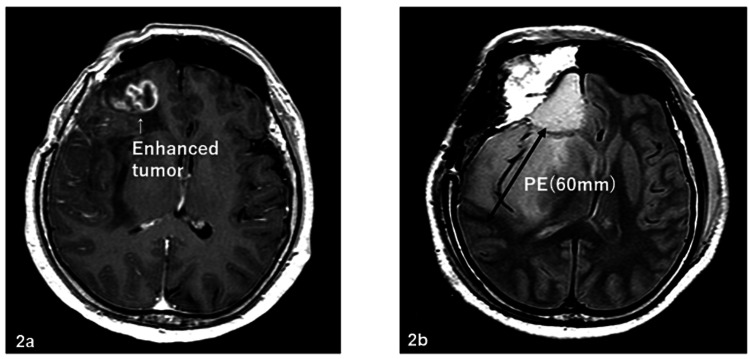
Supplementary MRI images – 2 2a: Gd-enhanced tumor before surgery; 2b: 60-mm PE after surgery MRI: magnetic resonance imaging; PE: preoperative edema
